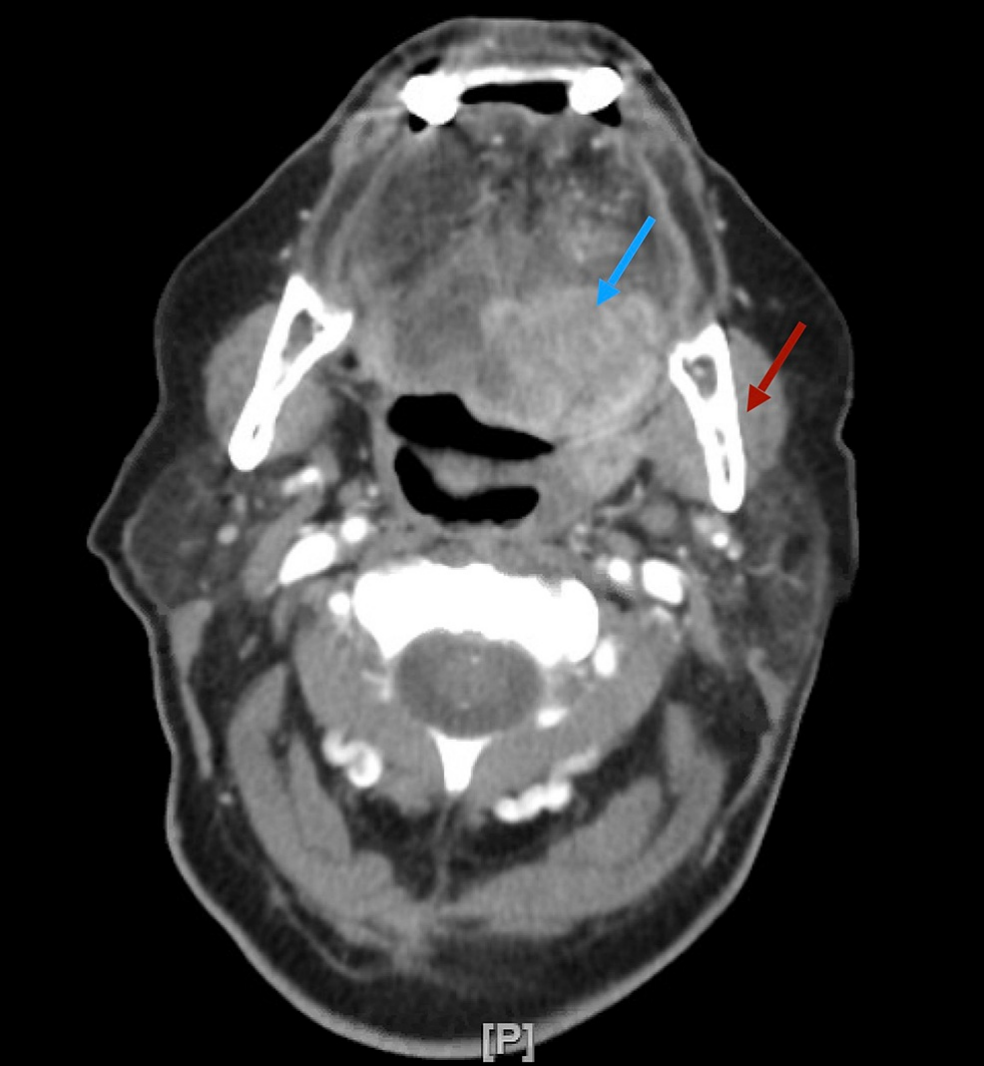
CT neck with contrast of an irregular, peripherally enhancing mass within the left sublingual (blue arrow) and submandibular space (red arrow) measuring up to 4.7 cm CT: computed tomography
